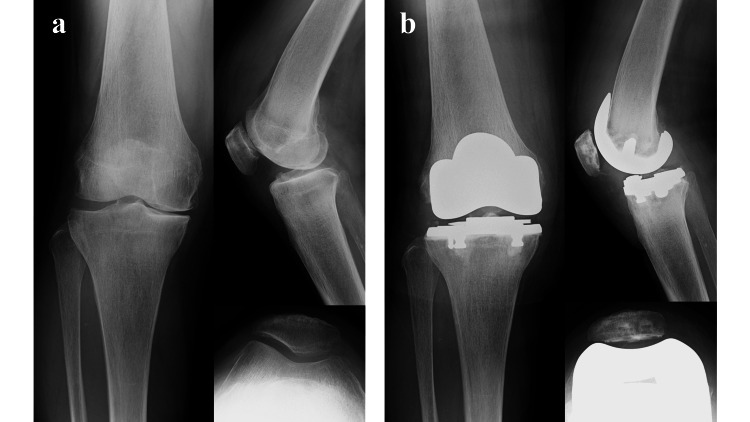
Preoperative (a) and postoperative (b) radiographs of a bicruciate retaining total knee arthroplasty (BCR-TKA).
